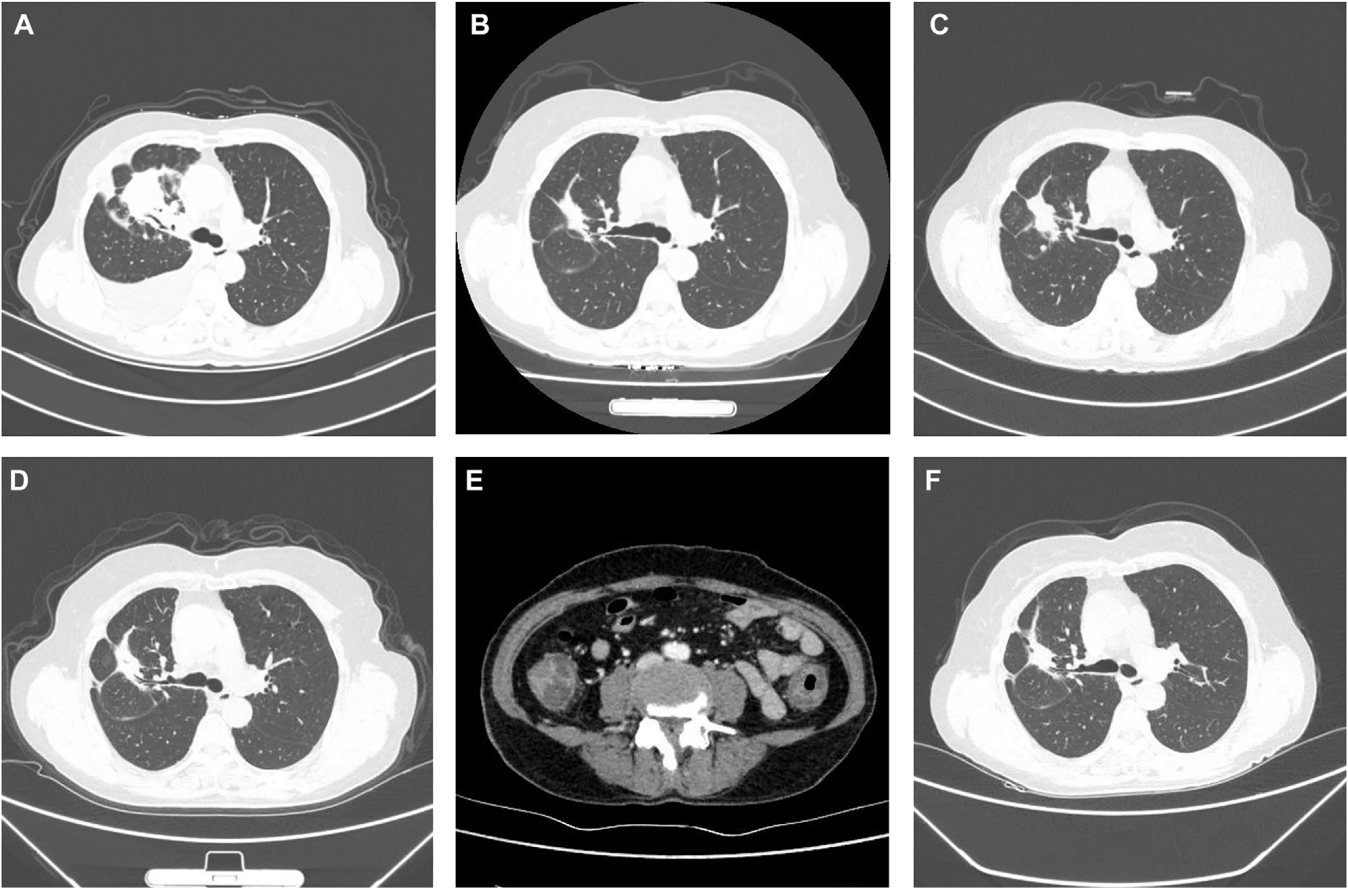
Clinical course according to CT scans. (A) Baseline CT scan at diagnosis in October 2019. (B) PR on icotinib in June 2020. (C) Disease progression in October 2020. (D) PR to osimertinib in January 2021. (E) Abdomen and pelvis CT in June 2021 showed that oedema had caused wall thickening of the colon. (F) SD on aumolertinib in November 2021.
